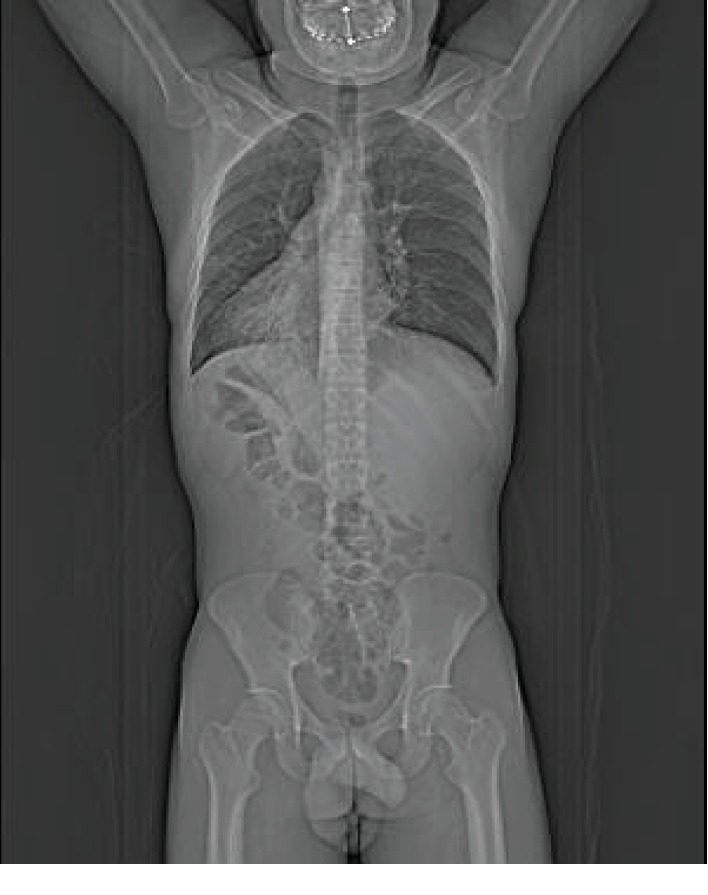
Multiplanar reconstruction in computed tomography scan highlighting the transposition of the great vessels and the abdominal and thoracic organs.
